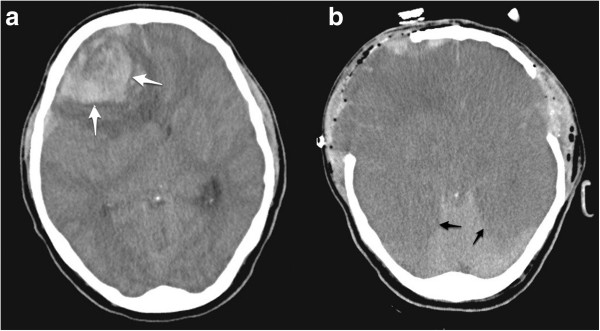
Computed tomography scan images. (a) Preoperative brain computed tomography scan showing an extensive intraparenchymal hemorrhagic lesion in the right frontal lobe (white arrows), with severe diffuse perilesional edema affecting the whole ipsilateral hemisphere, and compressing the ipsilateral lateral ventricle and causing significant mass effect. (b) Immediate postoperative computed tomography scan showing the low-density appearance of both the cerebral hemispheres, suggesting severe brain ischemia (black arrows).
